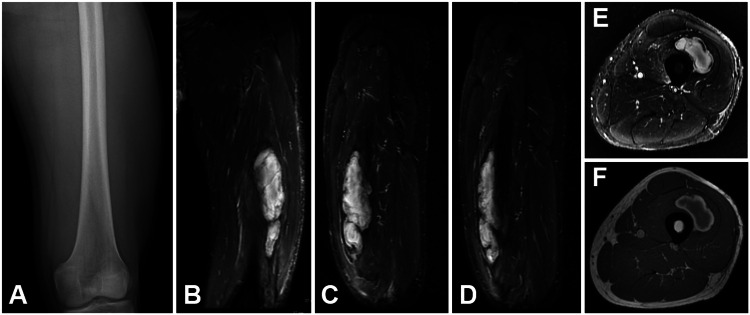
Imaging at presentation A: radiograph of the left femur at initial presentation without any signs of fracture; B: coronal MRI STIR sequence of left thigh showing large hematoma (15x3 cm); C,D: sagittal MRI STIR sequence of left thigh; E: axial MRI STIR sequence of left thigh; F: axial MRI T1 sequence of left thigh STIR - short tau inversion recovery
